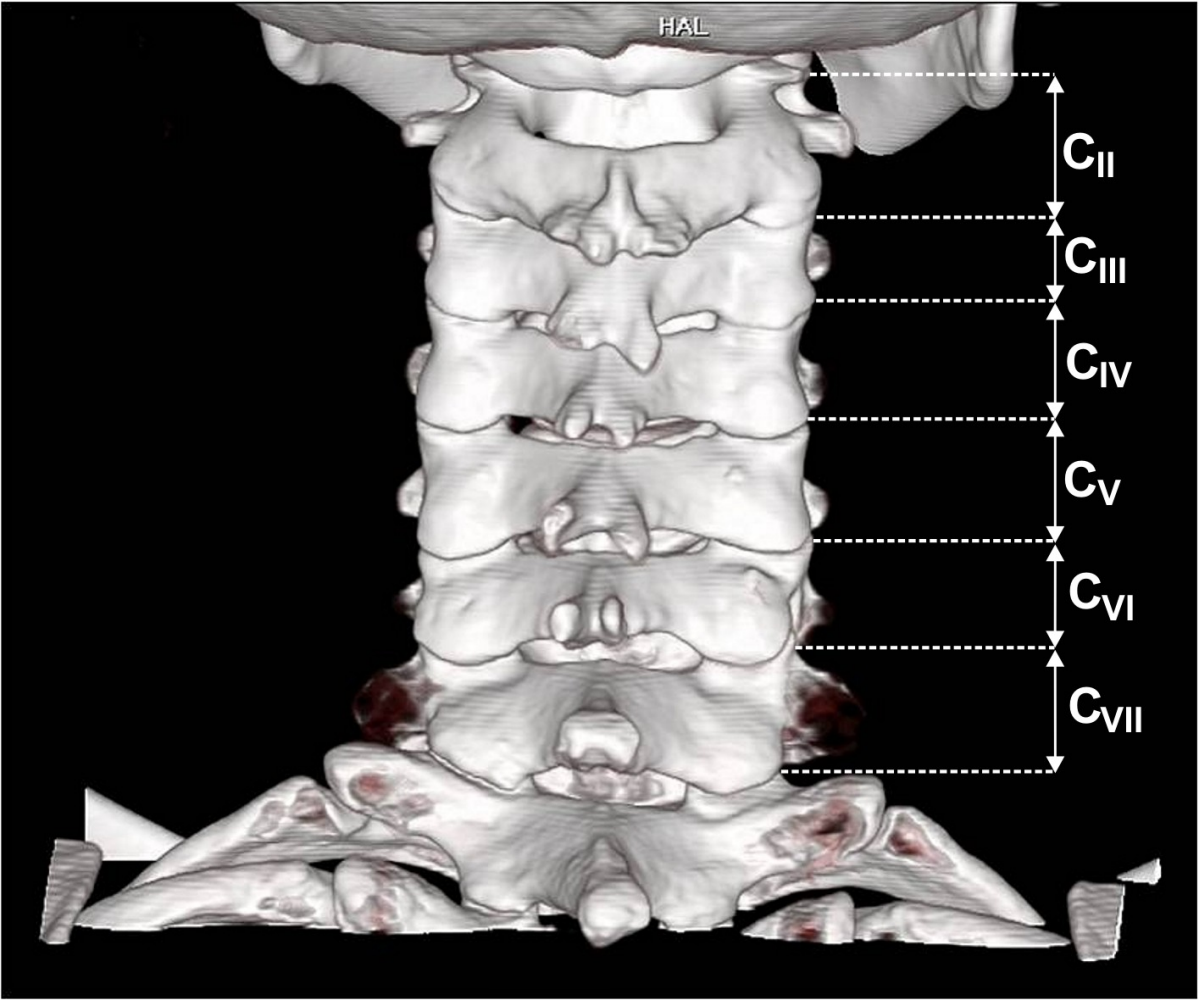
Three-dimensional volume rendering (VR) CT demonstrating bifid spinous processes in the cervical spine, level CII-CVII. Multidetector Computed Tomography (MDCT).
